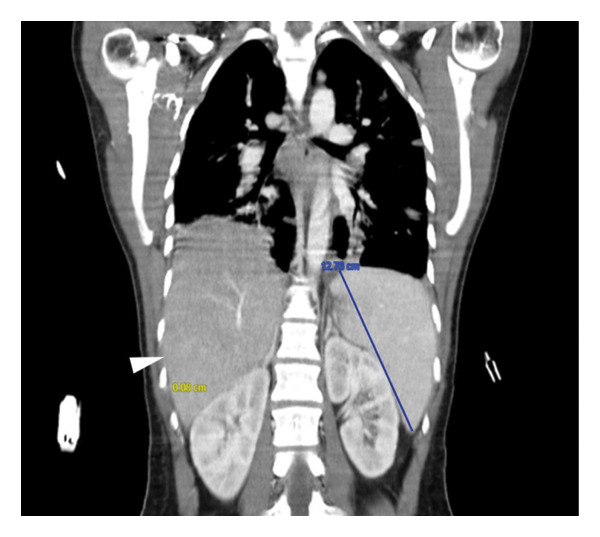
CT‐scan (coronal view) in which hepatosplenomegaly is seen (white arrow and blue mark).
